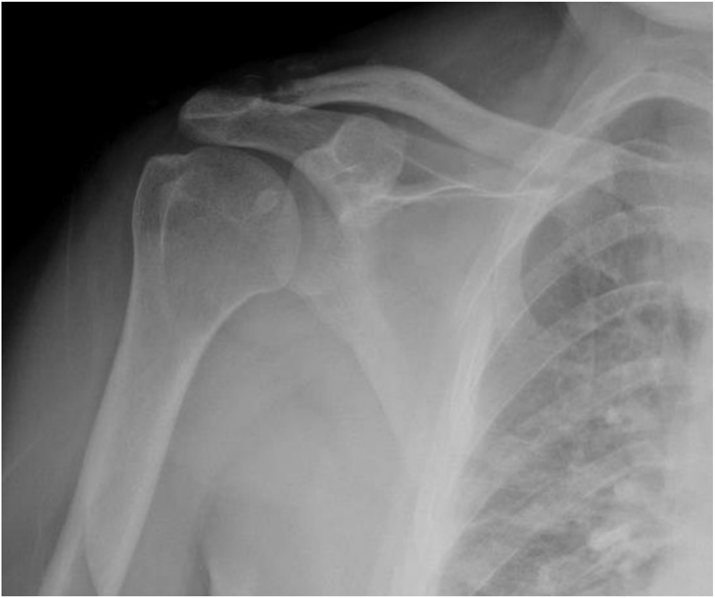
Final radiographs at 1 year showed good reduction of AC joint with minimal ossification, but no calcification or bony bar imaging or joint space narrowing.
